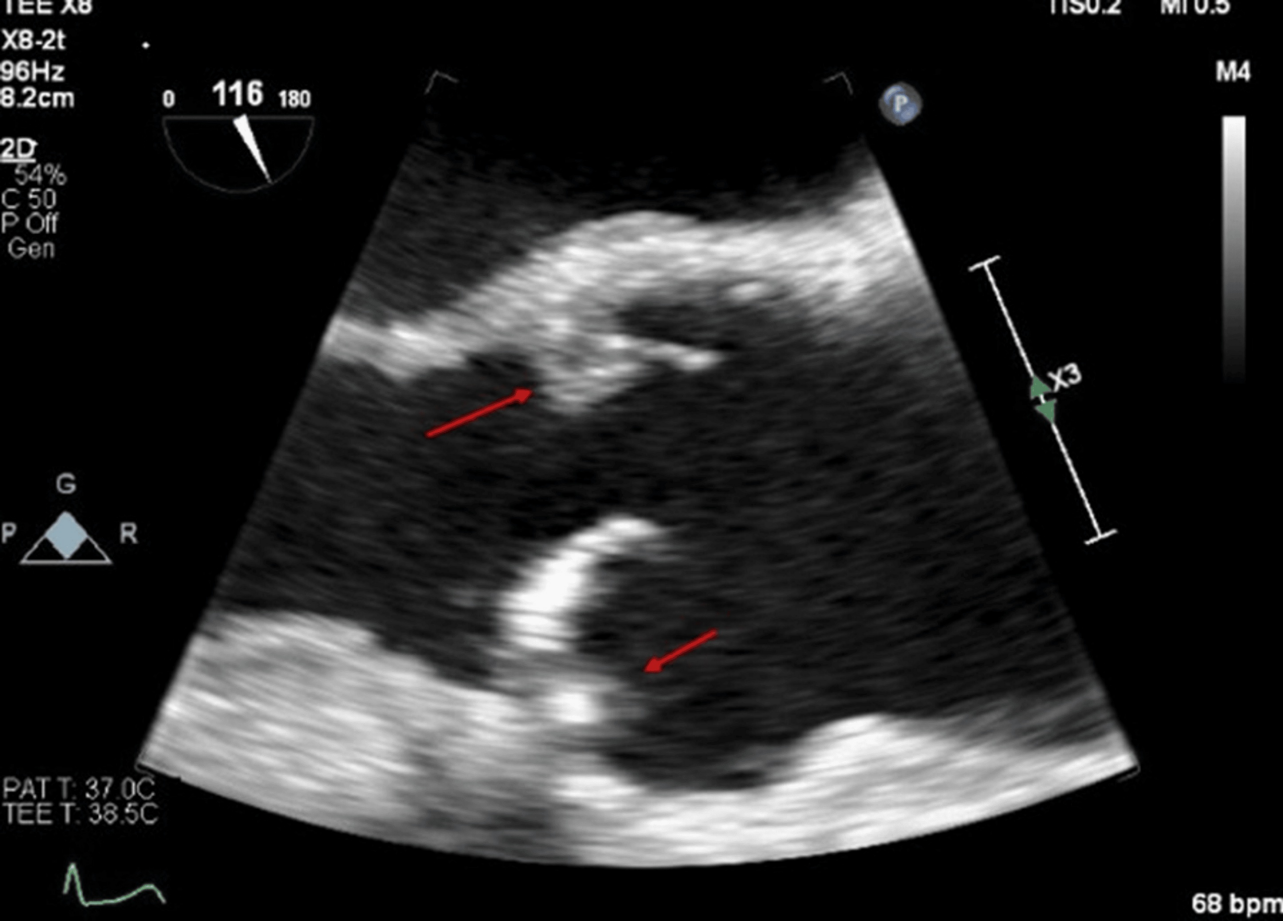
9Transesophageal echocardiograph showed fibroelastomas on atrioventricular (AV) leaflets and lipomatous hypertrophy of the interatrial septum noted on the right and noncoronary cusps with the largest measuring 0.1 cm by 1.0 cm denoted by the red arrows.
